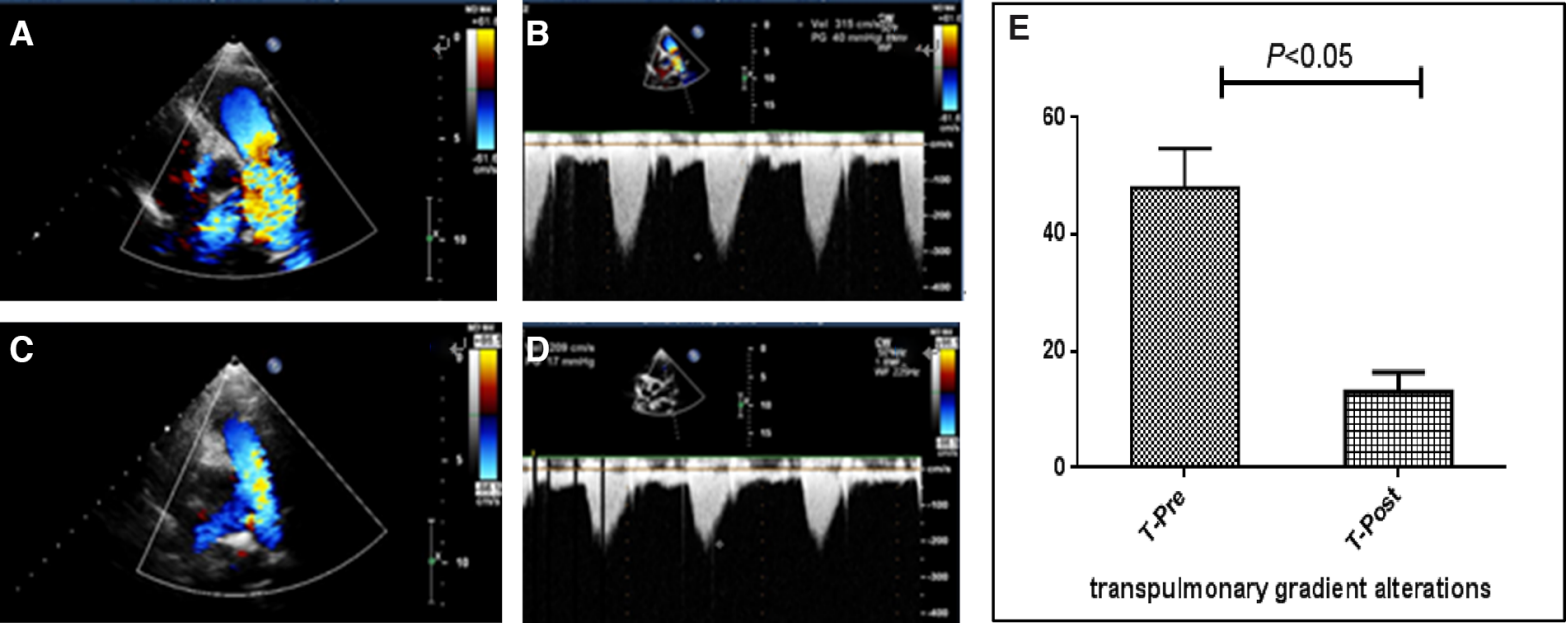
Images of echocardiography and pulmonary gradient (PG) changes in a patient with pulmonary stenosis (PS). Preoperation: (A) Parasternal short-axis view at the base with the main pulmonary artery and its branches displaying high-speed flow through the pulmonary valve with color flow Doppler. (B) Continuous-wave spectral Doppler showing full envelope spectral Doppler tracing; the measured velocity is approximately 3.1 m/s, or approximately 40 mmHg, as calculated by the simplified Bernoulli equation. Postoperation: (C) Color flow Doppler displaying smooth blood flow through the pulmonary valve. (D) Continuous-wave Doppler measuring the velocity to be approximately 2.0 m/s, or PG is calculated to be approximately 17 mmHg. (E) PG before and after percutaneous balloon pulmonary valvuloplasty is analyzed using a paired t-test, which revealing that PG decreased significantly.
